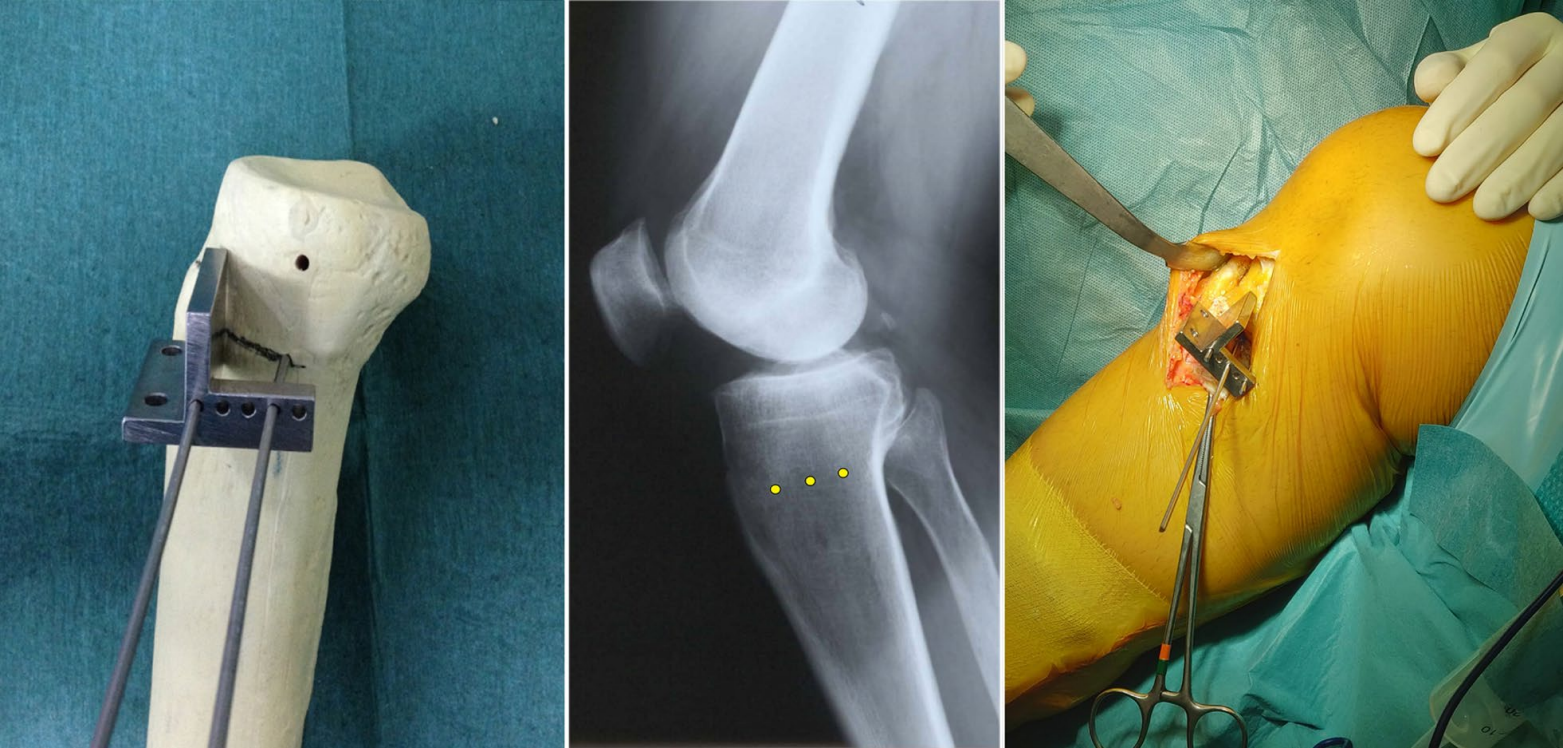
On the left, a demonstration of the second self-made cutting guide on a right tibia bone model. In the middle, a graphical representation of the three guide wires (yellow dots) on a lateral X-ray view of a right knee. On the right, the intraoperative findings of the self-made cutting guide positioning over the three wires, in a right tibia
